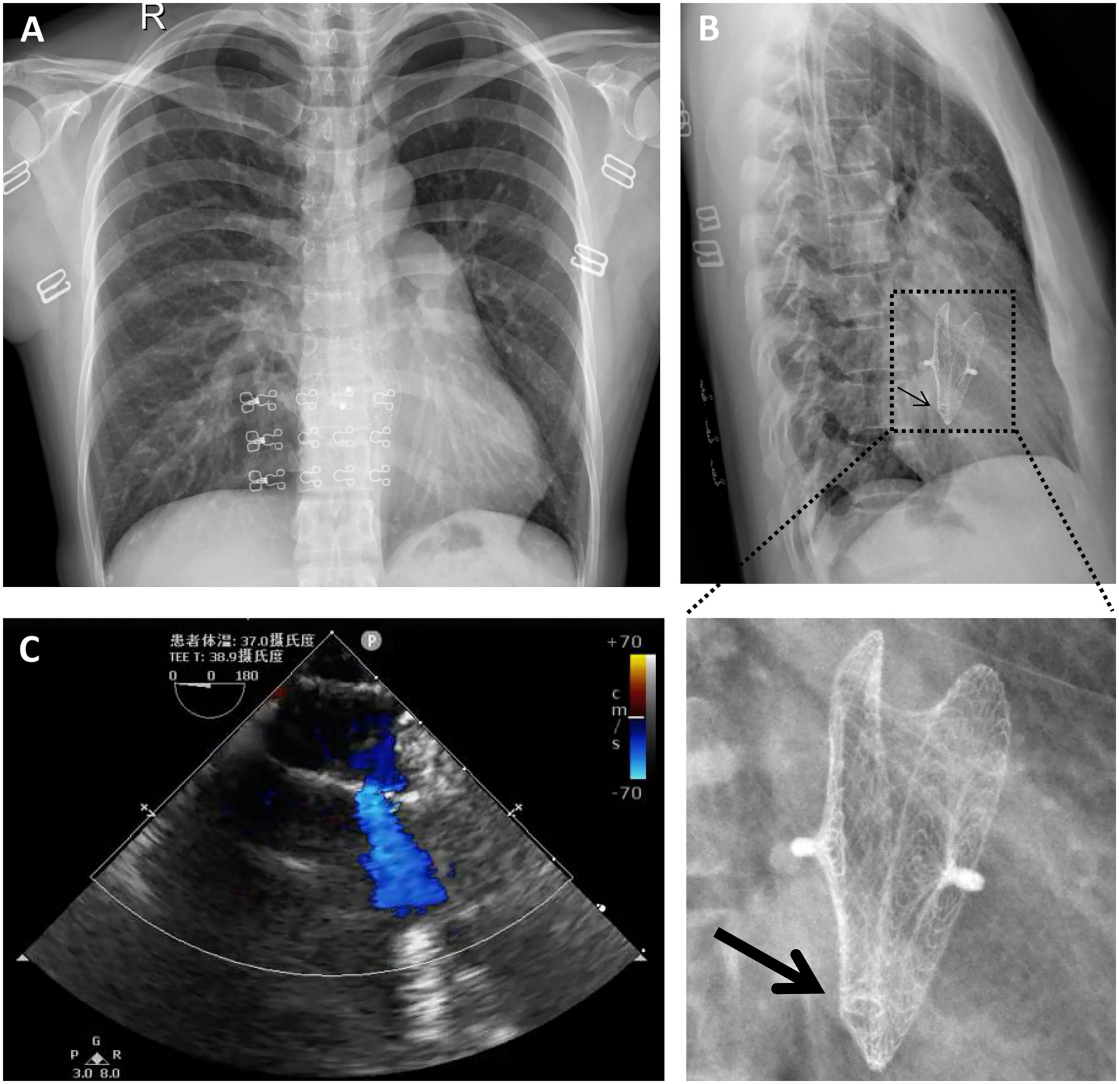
Chest radiography (A,B) and transthoracic echocardiography (C) preoperatively indicated that most parts of the closure were deformed, especially the left atrial side [(B), arrow]; a significant multi-bundle left-to-right shunt through the device and a small adhesion on the occluder was detected (C).
